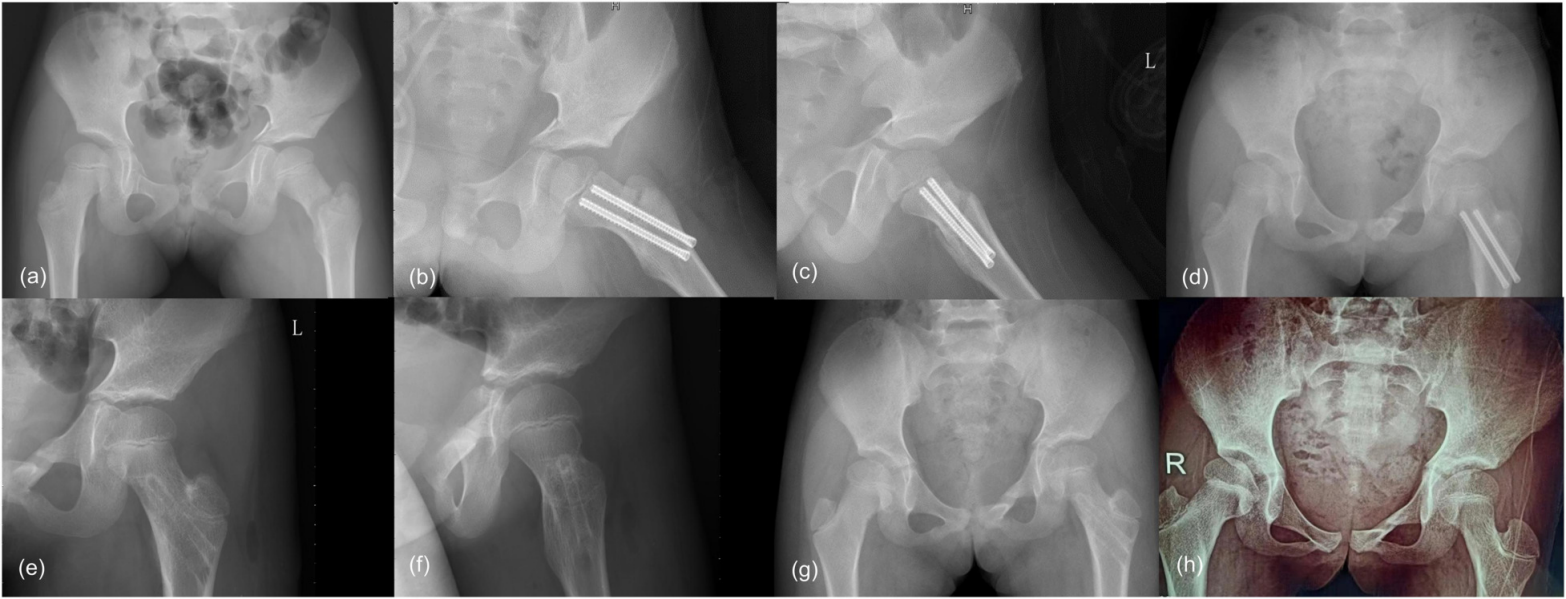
Radiographic outcomes of a 7-year-old girl with Delbet type III femoral neck fracture on the left side. (a) Preoperative pelvic radiograph. (b and c) Postoperative radiographs of the femoral neck on the first day after the operation. (d) Postoperative pelvic radiograph 1 year after the operation. (e and f) Radiograph of the femoral neck on the first day after hardware removal. (g) Radiograph of the pelvic 2 years after the initial surgery. (h) Radiograph of the pelvic 4 years after the initial surgery.
